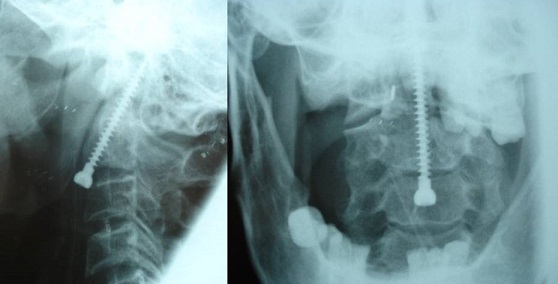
Post operative standard X-ray showing a patient who benefited from odontoid screwing
